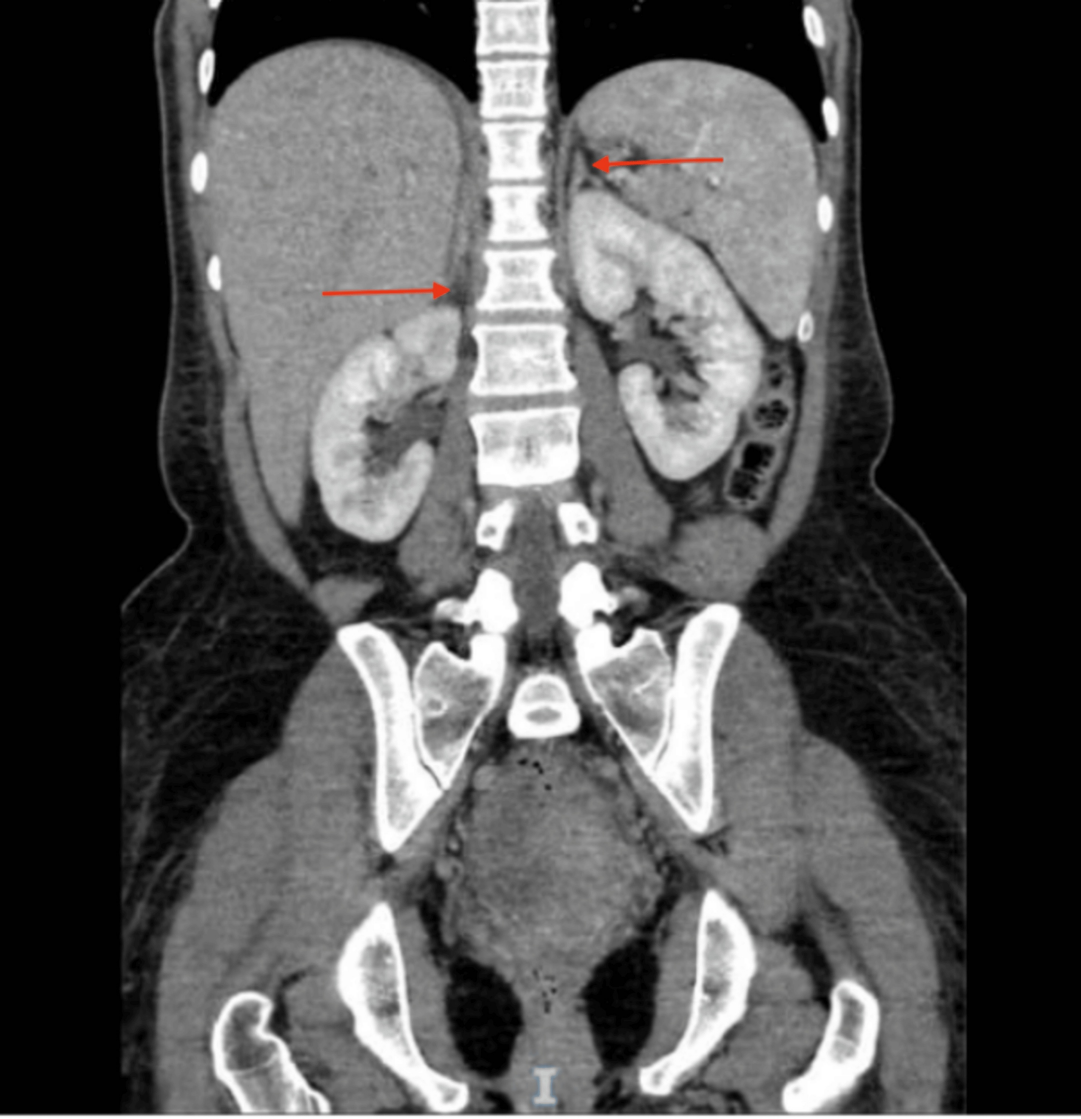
CT abdomen with contrast showing normal adrenal glands
